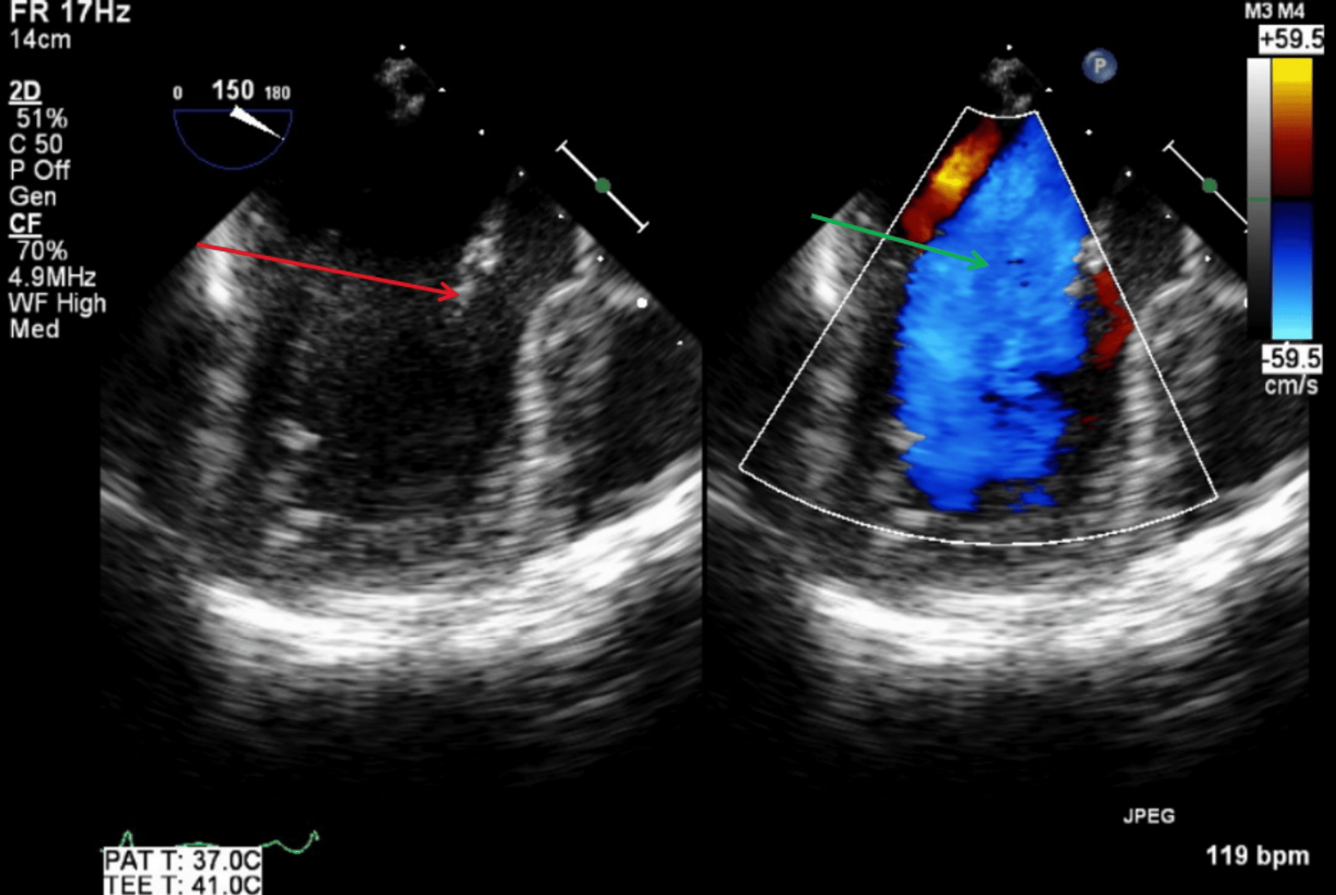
TEE mid-esophageal bi-commissural view with and without color Doppler during systole. The red arrow shows mitral valve with no visible vegetations. The green arrow shows no findings of mitral valve regurgitation with color Doppler. TEE: transesophageal echocardiography
